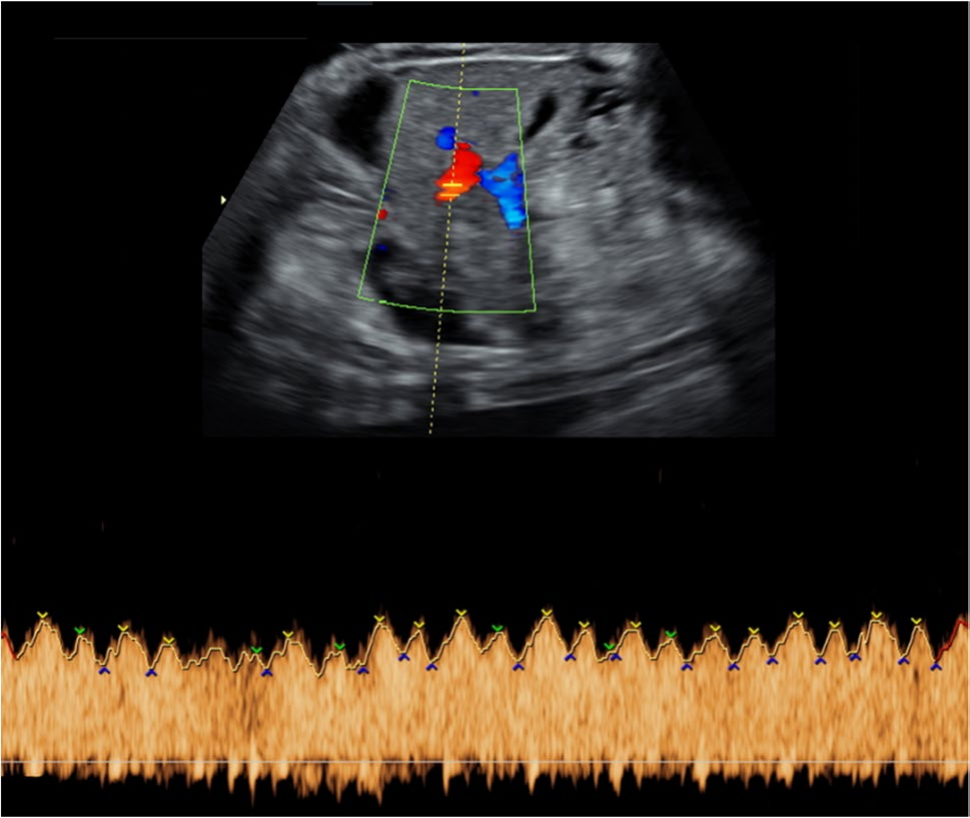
Case 9 at 32 + 6 weeks of gestation, CVPS 6 points (ascites, pleural effusions, generalized skin edema: 0 points, cardiothoracic area ratio = 0.2: 2 points, tricuspid valve regurgitation: 1 point, umbilical artery Doppler velocimetry with zero end-diastolic flow: 1 point, normal venous Doppler flow velocimetry: 2 points), yellow arrow: pleural effusion, white arrow: skin edema
